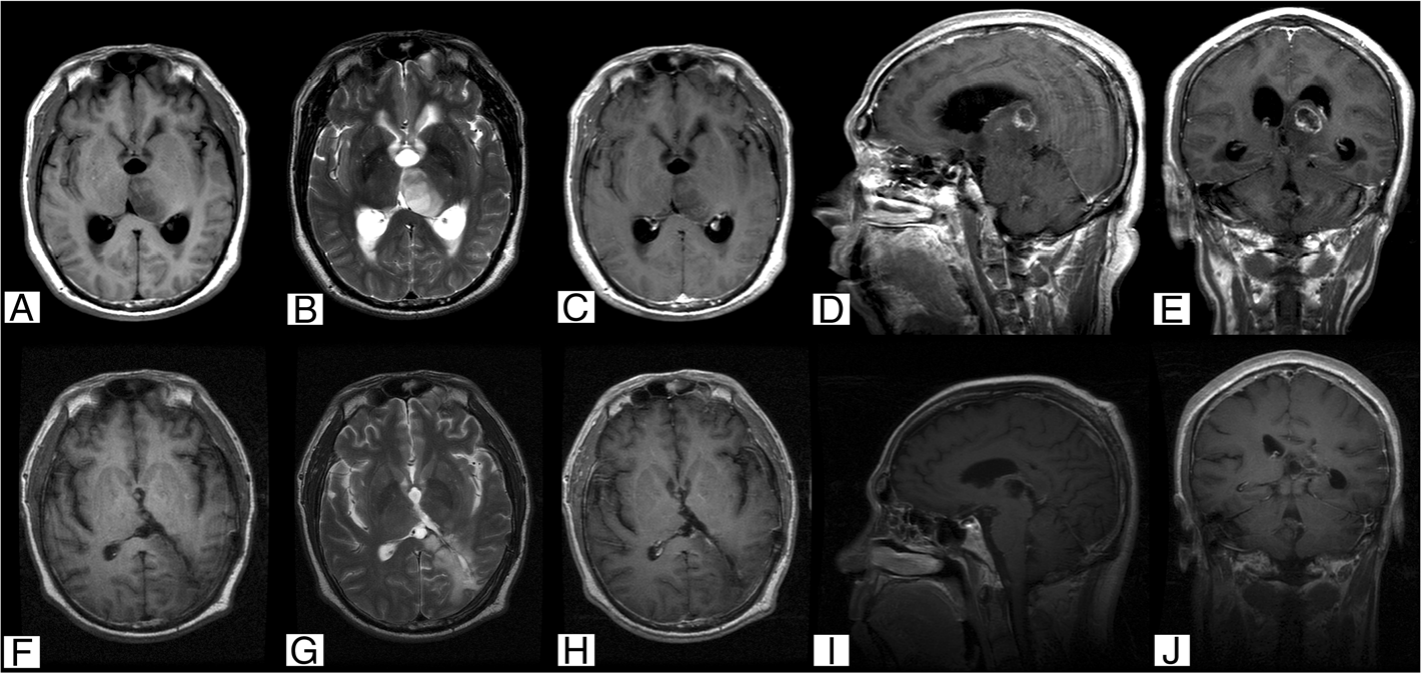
Case 1. Cranial MRI examination revealed unilateral thalamus glioma located in the left thalamus and midbrain with hypointense T1 (a) and hyperintense T2 (b) signals, which were heterogeneously enhanced after injecting contrast agent (c–e). Postoperative MRI confirmed subtotal resection (f–j). Pathological examination revealed a diagnosis of astrocytoma (WHO Grade II). Original magnification ×100
